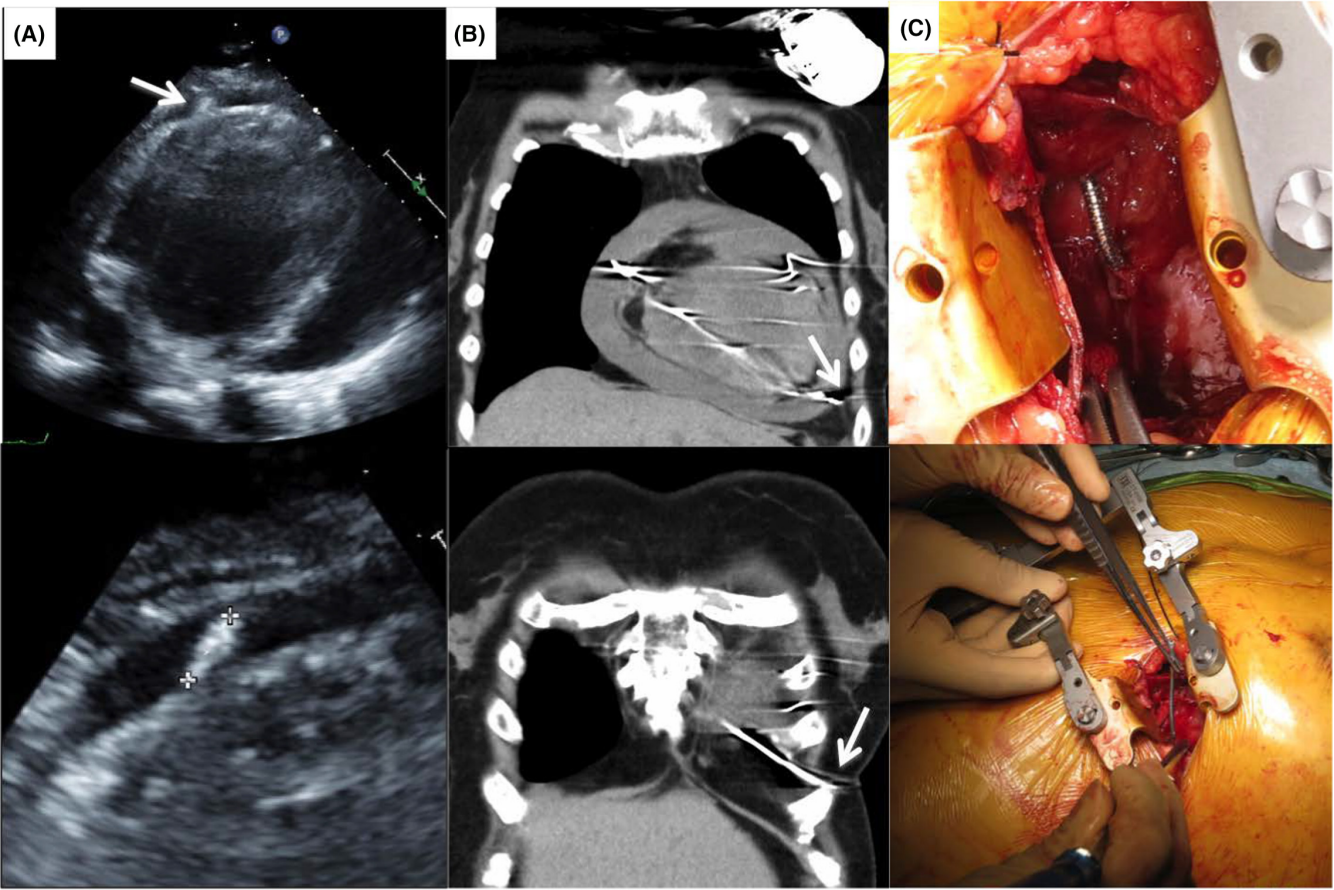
Investigation and confirmation of the cause of the patient's sudden complaints. (A) Echocardiogram showing severe pericardial effusion and the ICD lead (white arrow) extending beyond the ventricular apex. (B) Chest computed tomography image showing that the tip of the ICD lead (white arrow) had perforated the right ventricular wall and migrated beyond the epicardium. (C) Intraoperative photograph. Visual inspection confirmed that the ICD lead had perforated the right ventricular wall.
